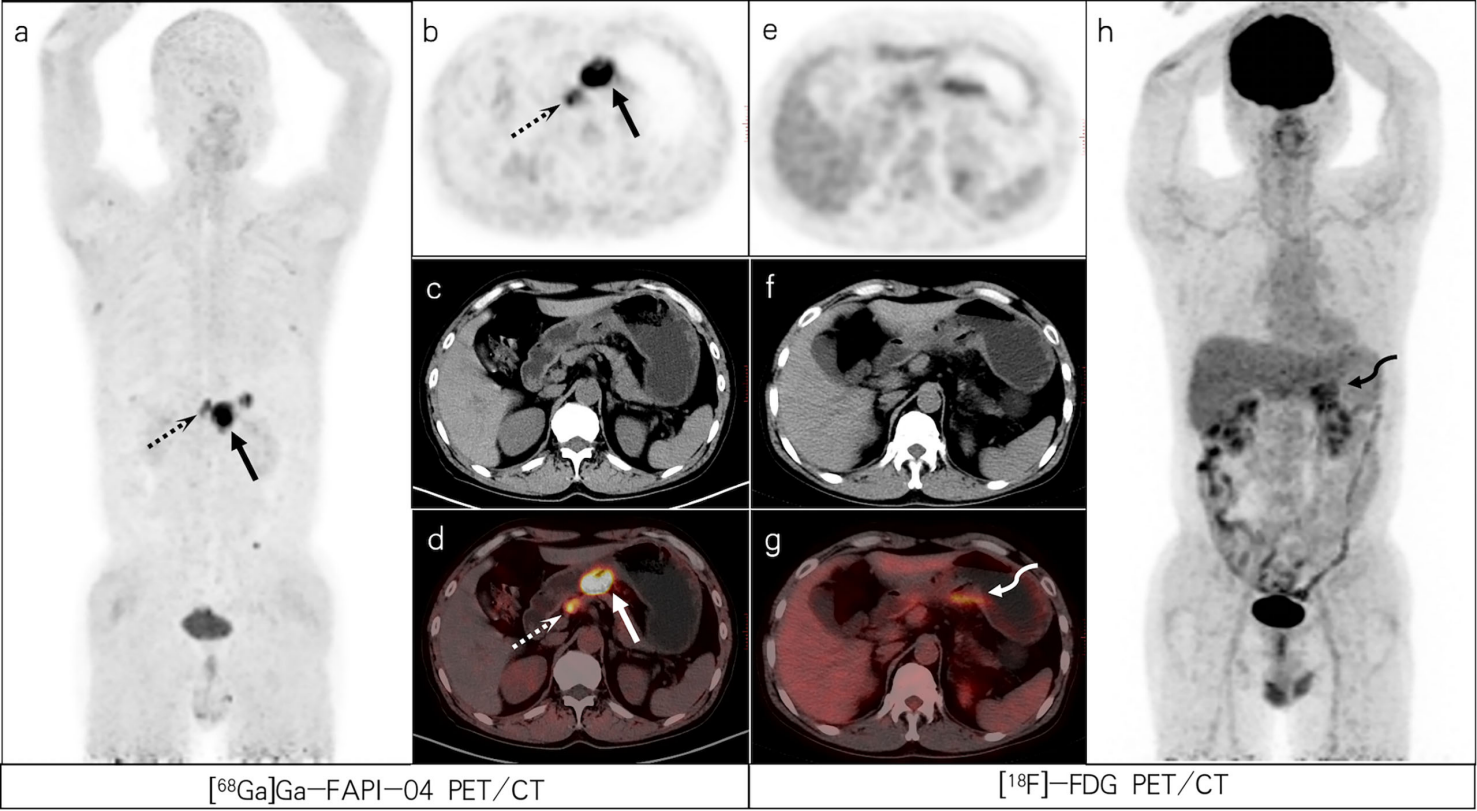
A 56-year-old male patient with poorly differentiated gastric adenocarcinoma based on pathological biopsy under gastroscopy. [^68^Ga]Ga-FAPI-04 PET/CT (A–D) revealed high uptake in the primary tumor (slender arrows, SUVmax=14.6) and lesser curved lymph nodes of the stomach (dashed arrows, SUVmax=8.7), but moderate uptake in the primary tumor (bent arrows, SUVmax=5.3) and negative uptake of lesser curved lymph nodes of the stomach were observed on [^18^F]-FDG PET/CT (E–H).
